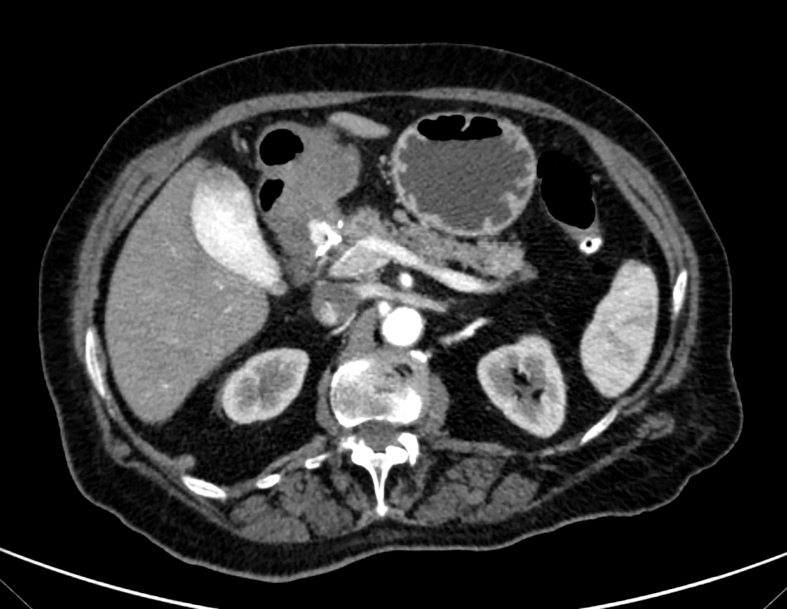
CT scan showing that the pseudoaneurysm was almost completely obliterated, with a residual small, vascularized spot of about 1 cm.
